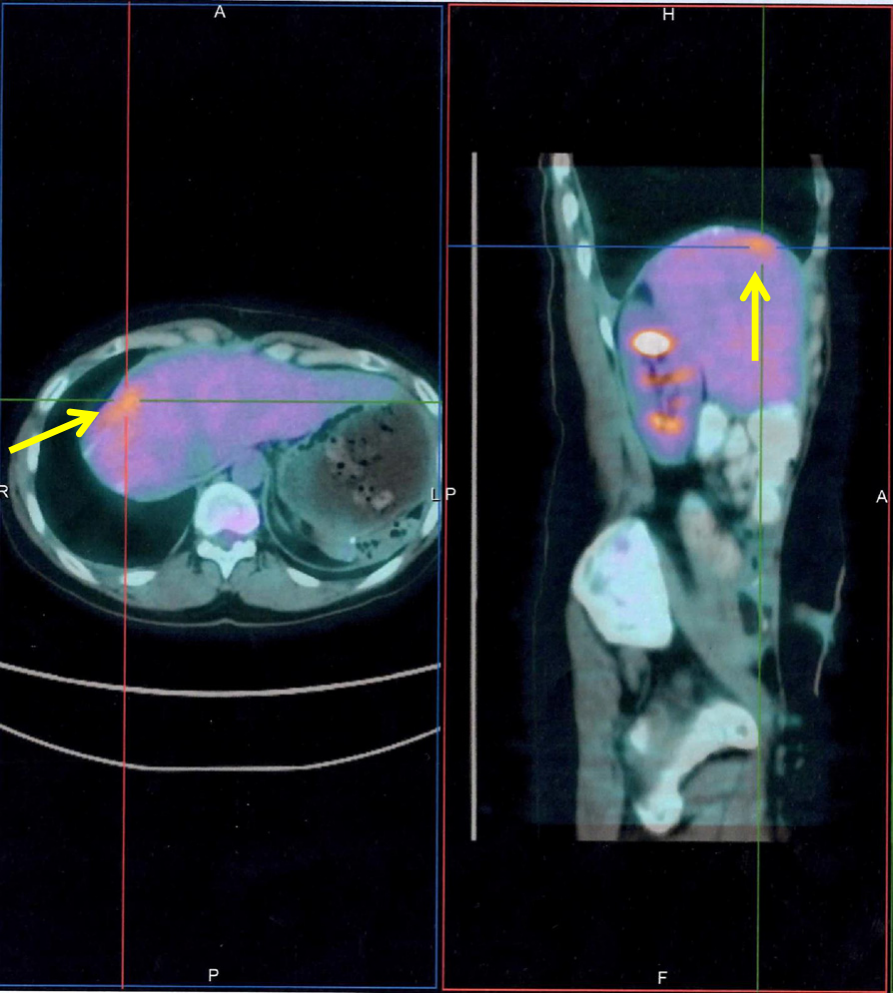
Positron emission tomography/computed tomography scan showing isolated high metabolic uptake (SUV: 4.2) in the right subdiaphragmatic region (arrows).
